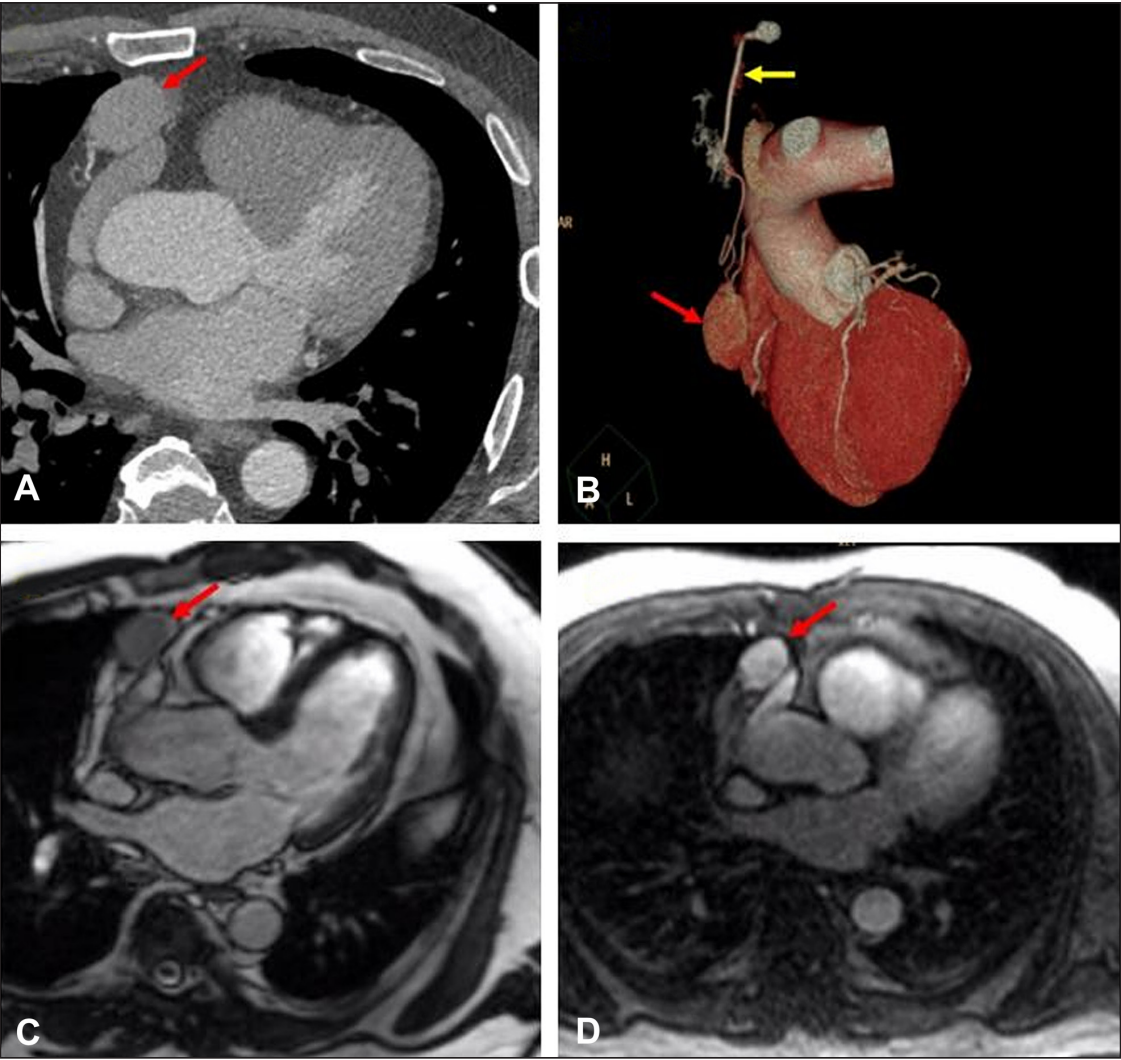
(A) Axial cross section on cardiac CTA showing the well circumscribed mass (red arrow) anterior to the right atrial appendage and adjacent to the pericardium. (B) A 3-dimensional reconstructed CT image of the mass with arterial supply from the right internal mammary artery (yellow arrow). (C, D) CMR images show the mass with significant first-pass perfusion and late gadolinium enhancement consistent with a highly vascularized structure.
